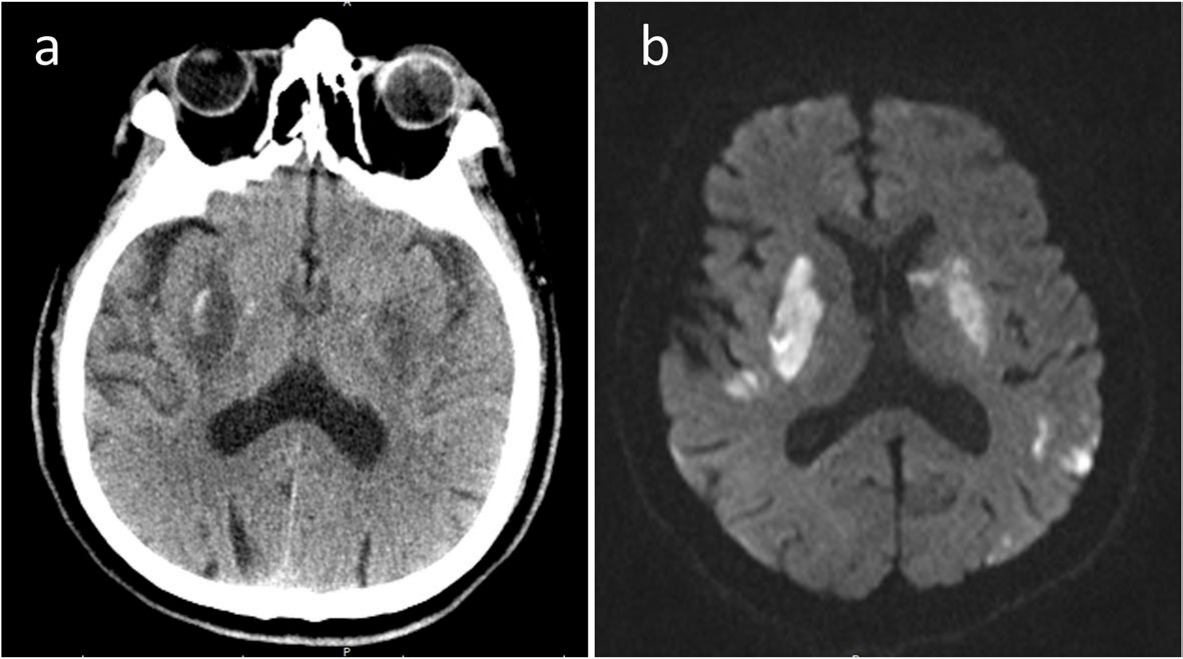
Postoperative imaging examination. a Brain CT showing a small amount of bleeding in the right basal ganglia. b Diffusion-weighted magnetic resonance axial imaging showing new cerebral infarction in the bilateral basal ganglia and bilateral cortex
